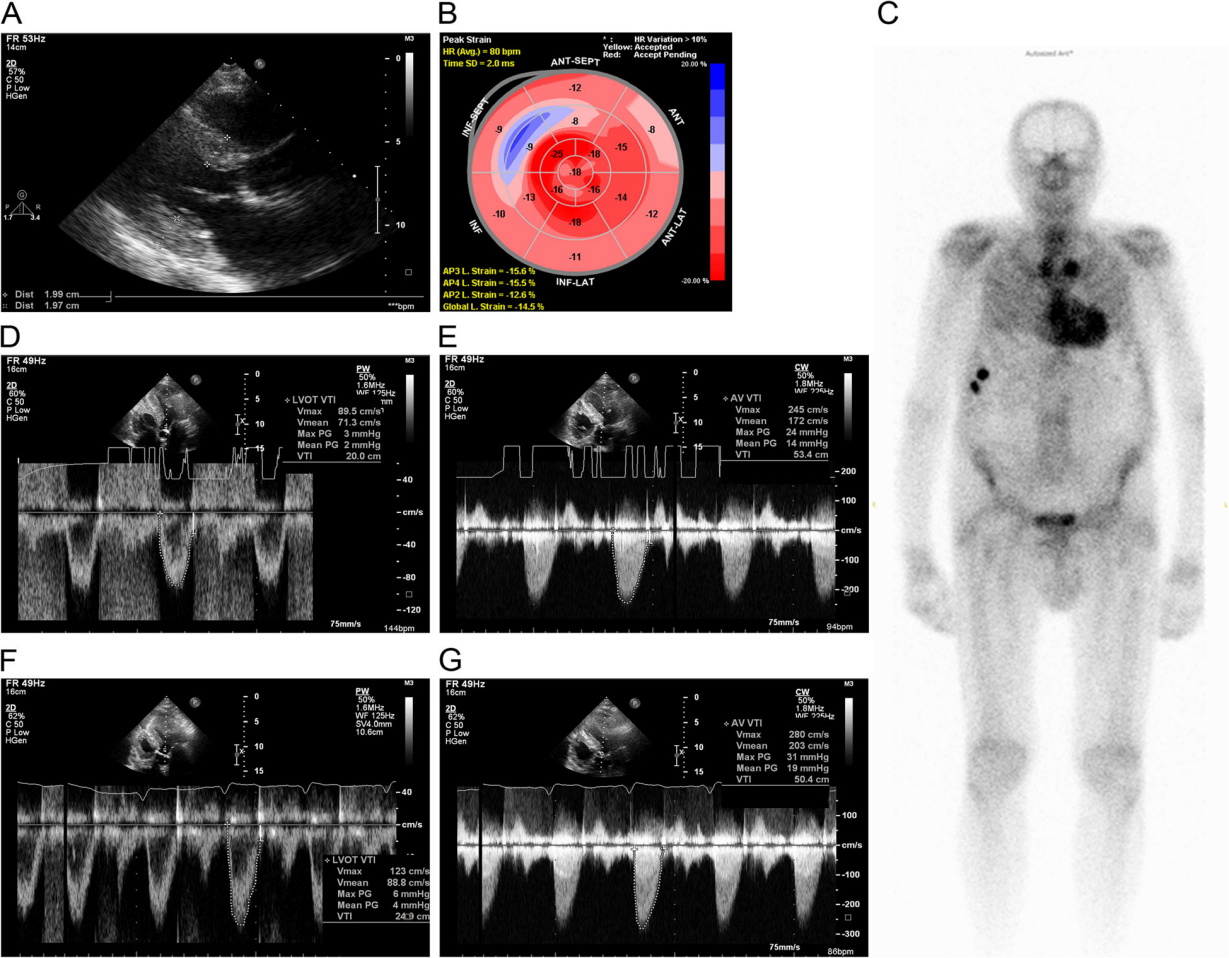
Images of a patient with wtTTR cardiac amyloidosis and low flow-low grade, pseudo-severe aortic valve stenosis. LVEF was 51%. a: Transthoracic echocardiography, parasternal long axis view. Septum and inferior wall are 20 mm thick at end-diastole. b: Bull’s eye image of the left ventricular longitudinal strain. Typical apical sparing. c: Pyrophosphate isotope scan, with significant take up of the tracer in the heart: Perugini score 3. d-g: PW and CW Doppler images of the left ventricular outflow tract at rest and at low dose dobutamine stress test. D: Resting PW Doppler, e: Resting CW Doppler. Resting calculated AVA: 0.54 cm2/BSA. f: PW Doppler at dobutamine test. g: CW Doppler at dobutamine test. Significant elevation in SV and AVA at dobutamine test. Calculated AVA at dobutamine test: 0.76 cm2/BSA
